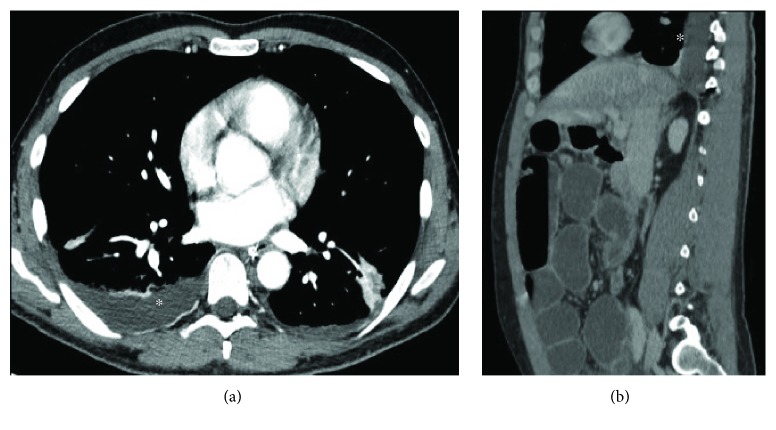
A 43-year-old man with Crohn's disease subjected to previous surgical ileal resection. Asterisks (^∗^) show unilateral pleural effusion during acute abdomen due to intestinal obstruction. Axial CT study (a) and sagittal reconstruction (b).
